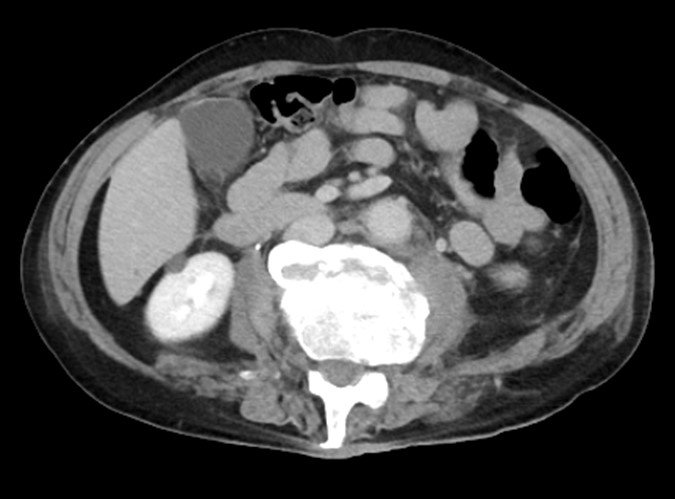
A contrast computed tomography scan taken 3 weeks after the injury showed that the hematoma almost disappeared, and there was no bleeding from the inferior vena cava.
